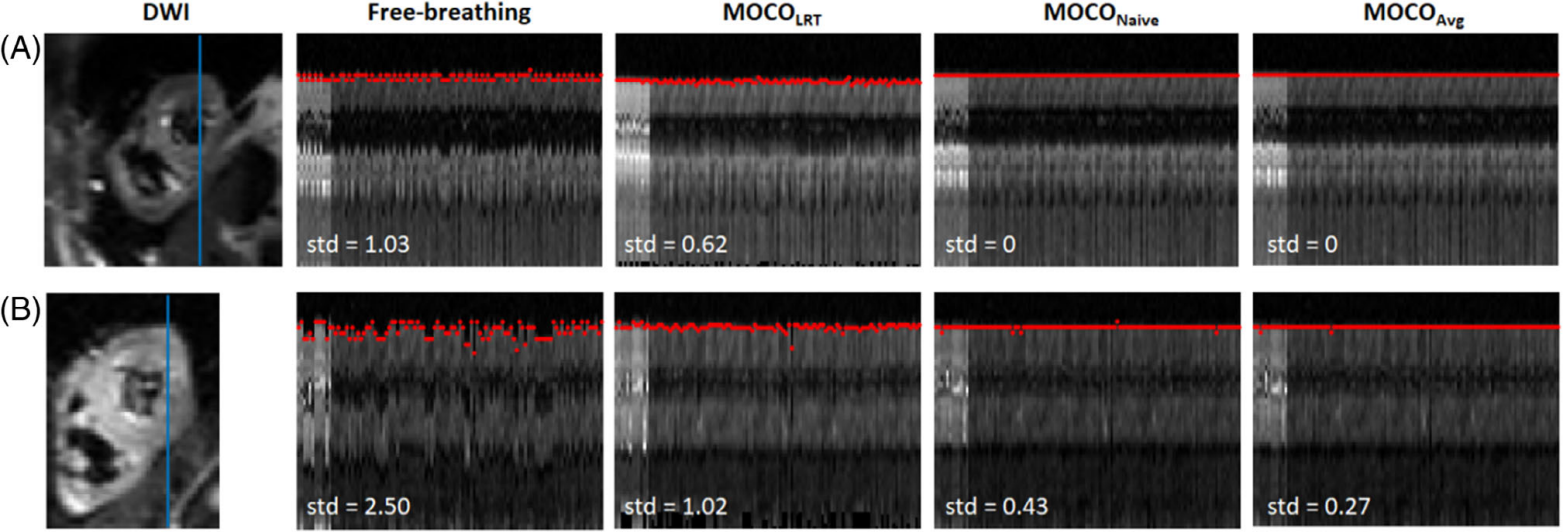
A line across the heart (indicated by the blue line in the DWI image) in two healthy volunteers is plotted across all frames for free‐breathing data, and images resulting from motion correction (MOCO) methods MOCO_LRT_, MOCO_Naive_, and MOCO_Avg_. Epicardium pixels are labeled with red circles. SD of the epicardium pixel positions across all image frames are also labeled. (A) shows a case with less motion before MOCO compared with (B).
